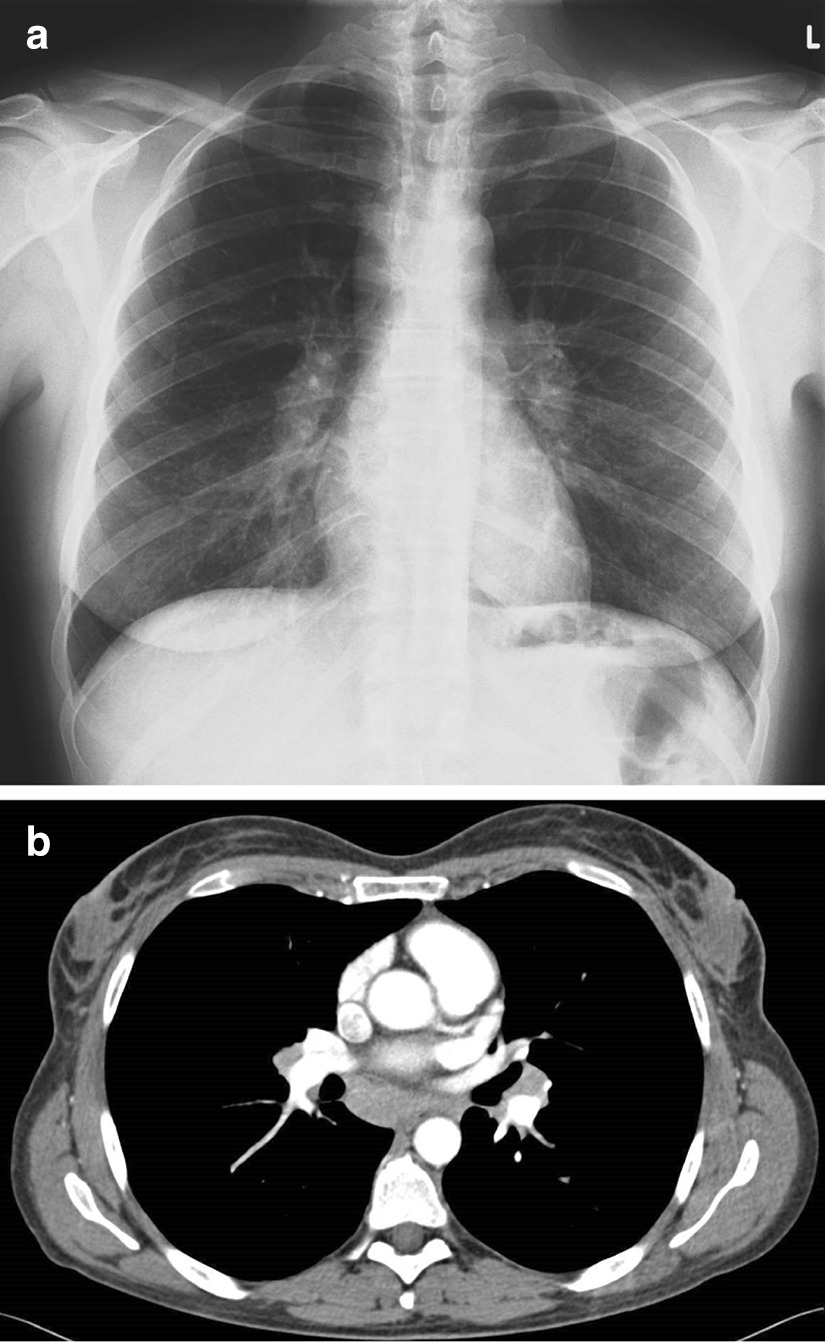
a Chest radiograph showing infiltrative minimal abnormalities in the left upper field, and bi-hilar and mediastinal masses suggestive of sarcoidosis. b Computed tomography scan image confirming bi-hilar lymph node enlargement
